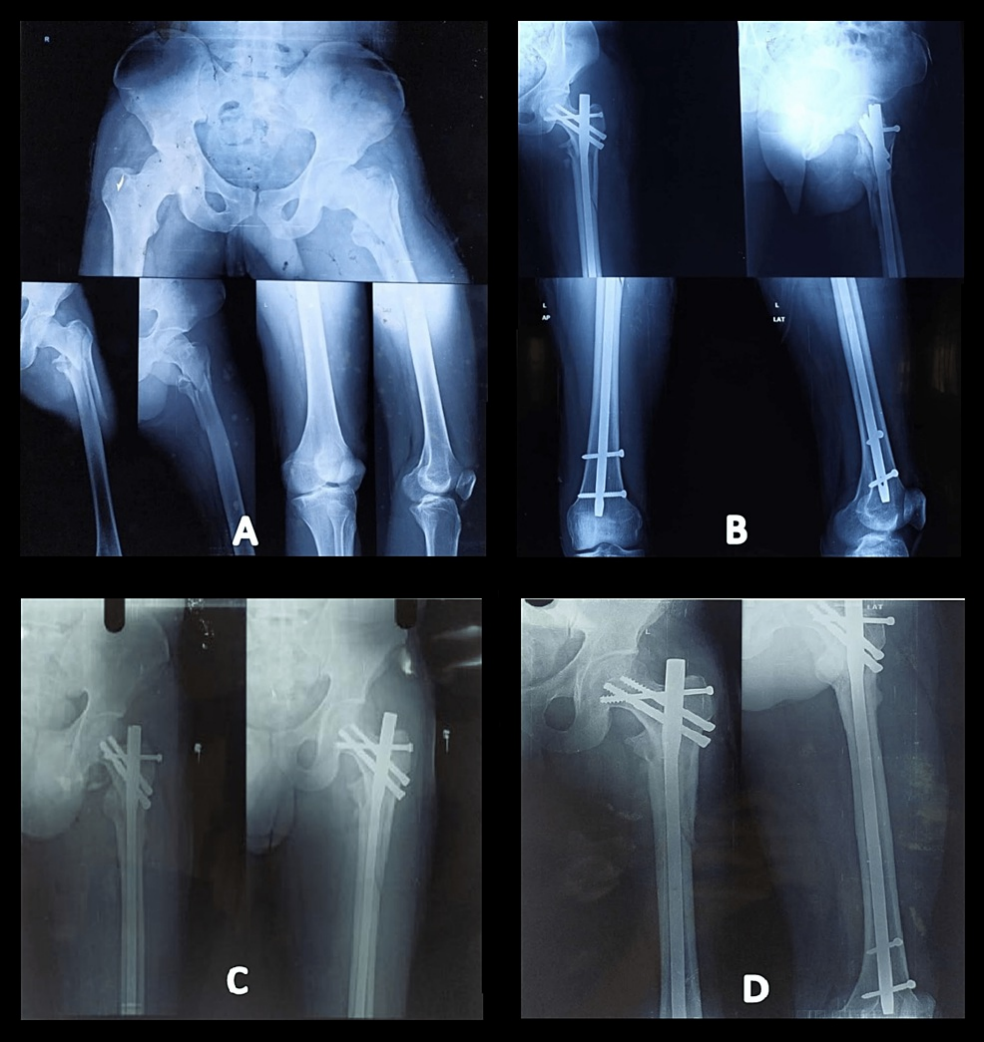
Serial follow-up radiographs of a 31-year-old male with unstable intertrochanteric fracture (AO/OTA type 31-A2.3) of the left side managed by proximal femoral nail augmented with screw A: Pre-operative radiographs showing the sustained fracture pattern
